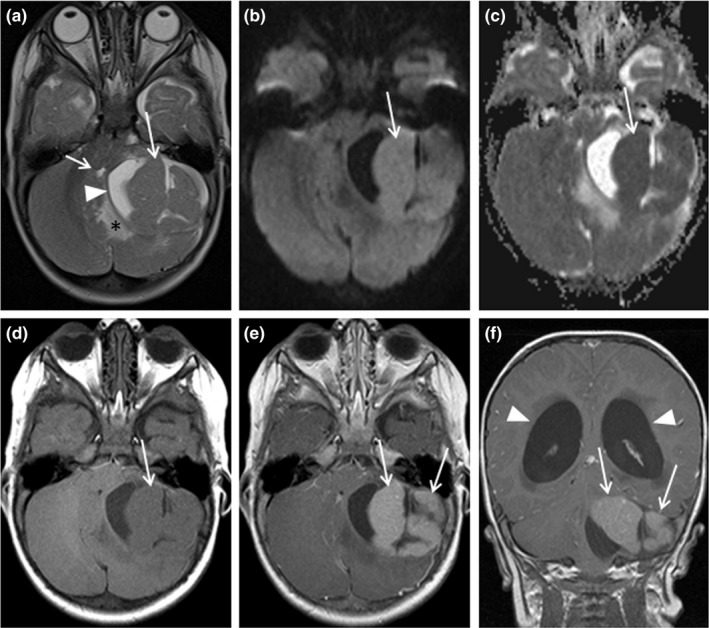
Brain MRI showing a tumor of the posterior fossa of a 14‐month‐old girl. T2‐weighted axial image (a) demonstrates a large cerebellar intraparenchymal hemispheric tumor with T2‐hyperintense cystic (arrowhead) and T2‐hypointense nodular solid (long arrow) components causing compression of the 4th ventricle (short arrow) and surrounding edema of the cerebellum (asterisk). Restricted diffusion of the solid components (b and c, arrows) suggesting highly cellular tumor is demonstrated on diffusion‐weighted imaging (b) with apparent diffusion coefficient (ADC) map (c). The solid component is slightly hypointense compared to cerebellum on native T1‐weighted image (d, arrow) but it enhances intensely with gadolinium contrast media showing nodular arrangement on contrast‐enhanced T1‐weighted axial (e) and coronal (f) images (arrows), which is indicative of desmoplastic nodular medulloblastoma. Lateral ventricles are dilated due to the compression of the tumor on the 4th ventricle (f, arrowheads). MRI, magnetic resonance imaging
